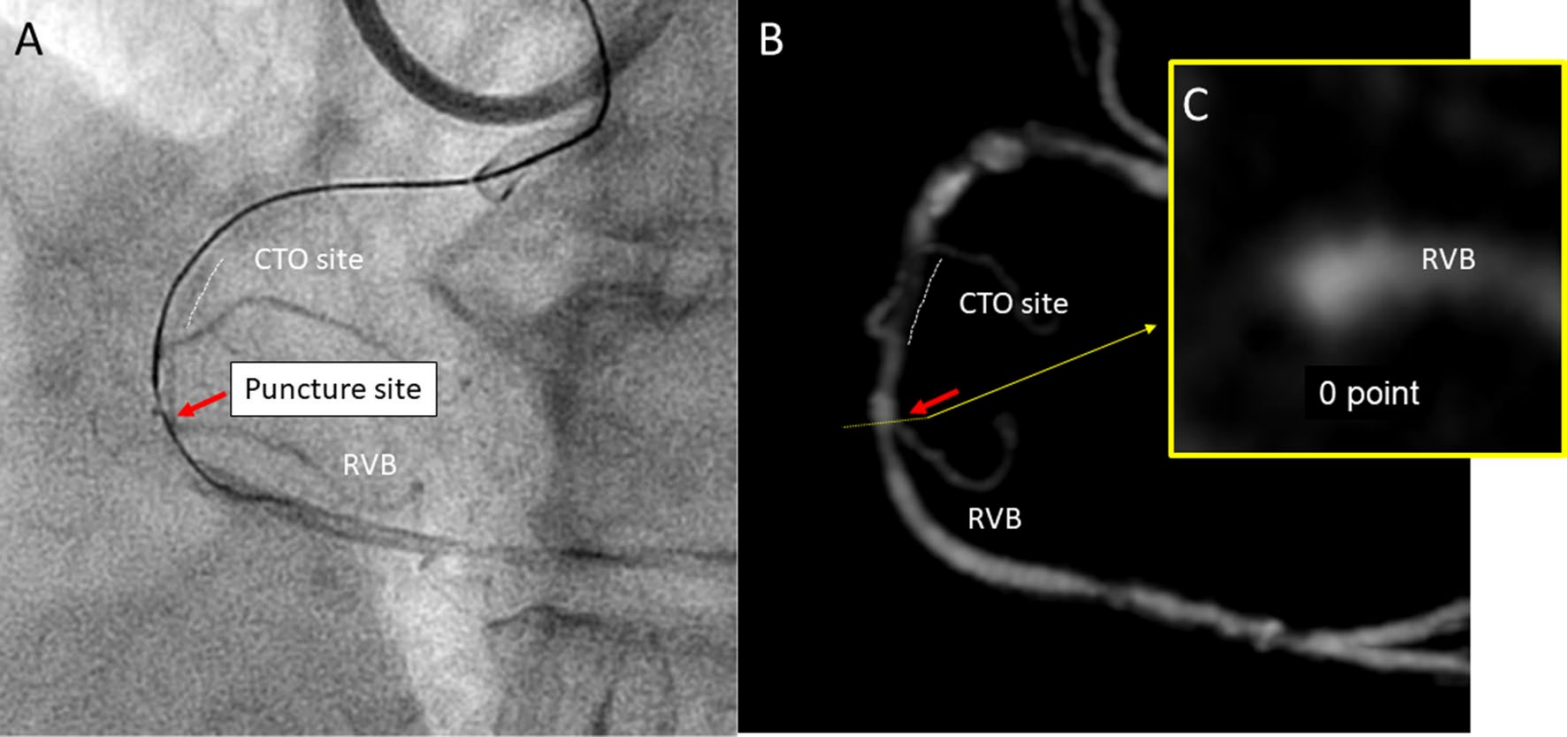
Example of computed tomography (CT) score evaluation at puncture site. Case with chronic total occlusion (CTO) in right coronary artery succeeded by antegrade dissection re-entry (ADR) procedure. a Angiography when stick with confienza8-20 and stingray balloon was performed. Red arrow indicates the puncture point. b Curved image of coronary CT. Red arrow indicate puncture point beside the right ventricular branch selected according to the percutaneous coronary intervention angiographic image. c CT score was calculated using by cross-sectional image at the site
